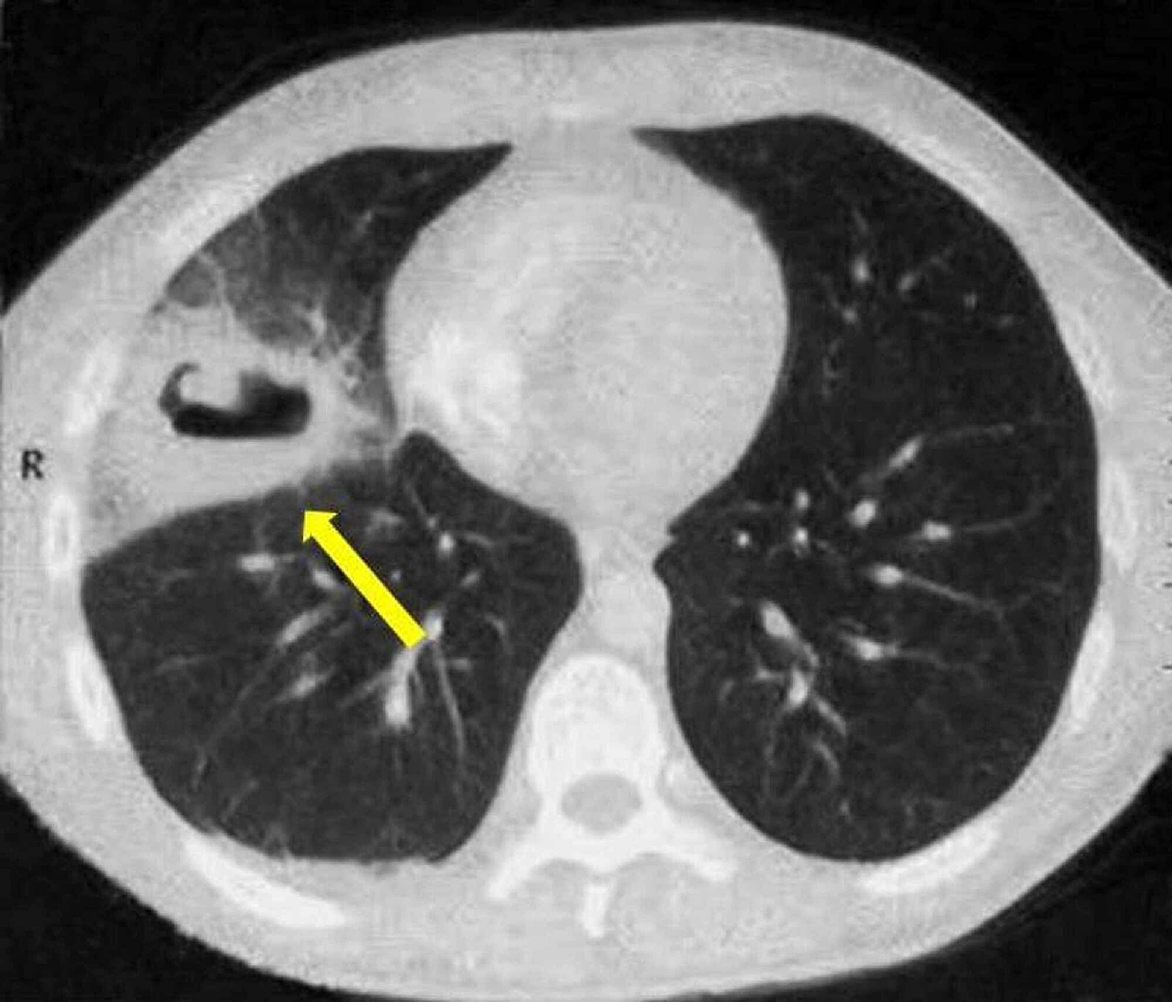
Chest-CT with contrast agent, axial view, showing a rounded lesion with a thick wall (arrow) in the right lower lobe.
